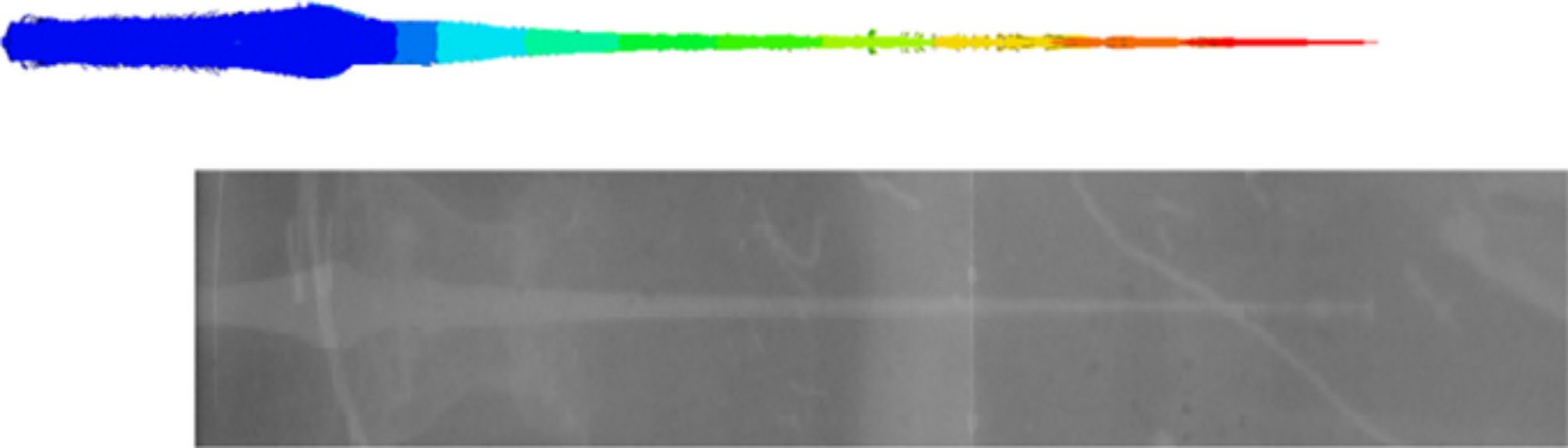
Comparison of jet profiles (numerical and x-ray trial) nearly at the same time.
